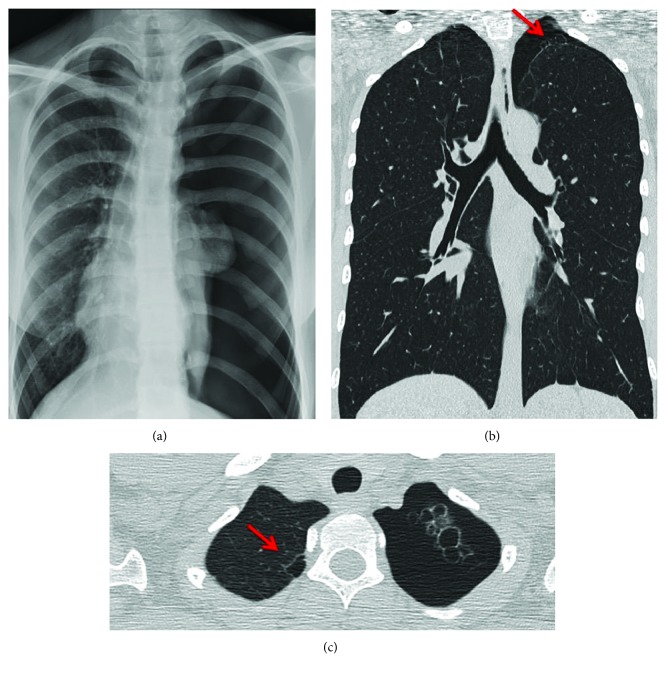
Lung imaging. (a) Chest X-ray. A total collapse of the left lung was showed. (b, c) Thoracic CT. A bullous pulmonary dysplasia was noted (the bullous lesion is indicated with an arrow).
